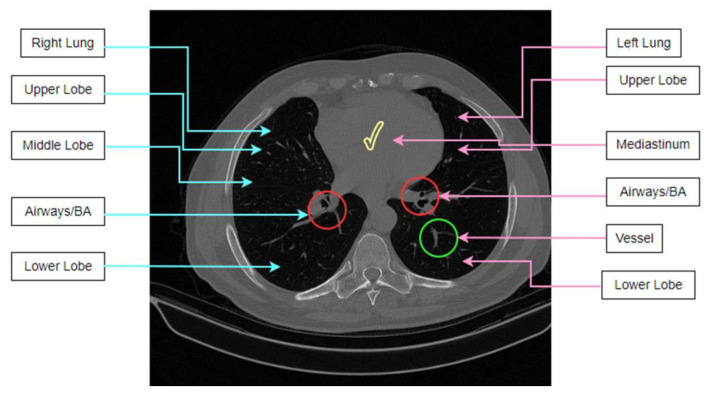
Anatomical structures in a lung CT scan.
